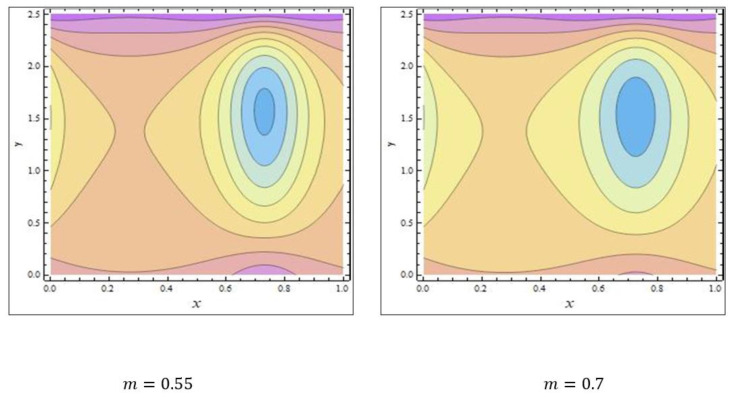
The streamlines of m. The values of the other parameters were $N_{CT}=0.3,\;N_{TC}=0.7,\;G_{rc}=0.4,\;G_{rF}=0.1,\;G_{rt}=0.5,\;N_{t}=0.7,\;M=3.5,\;\phi =\frac{\pi }{6},\;\Gamma =0.8,\;\beta =0.7,\;Q=2.37$.
